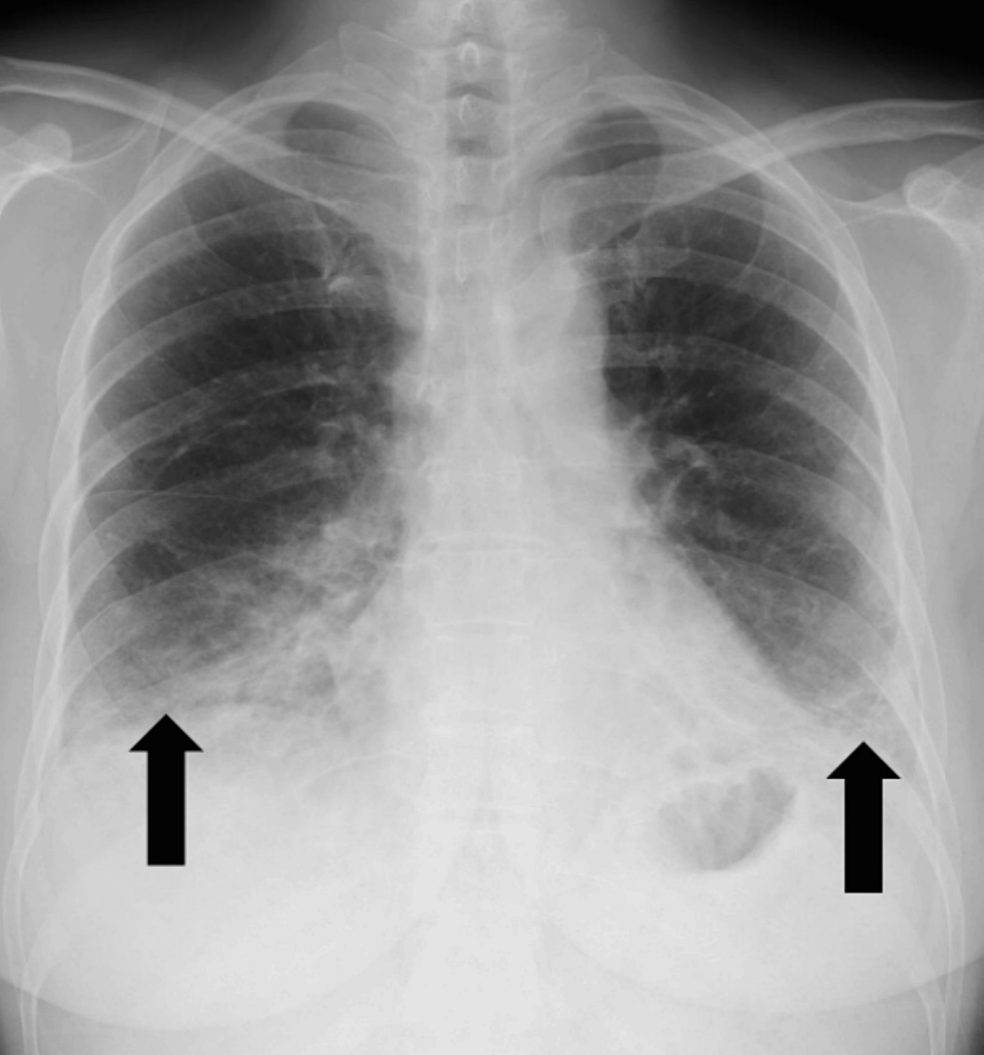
Plain chest radiograph on admission showing interstitial changes (arrow)
